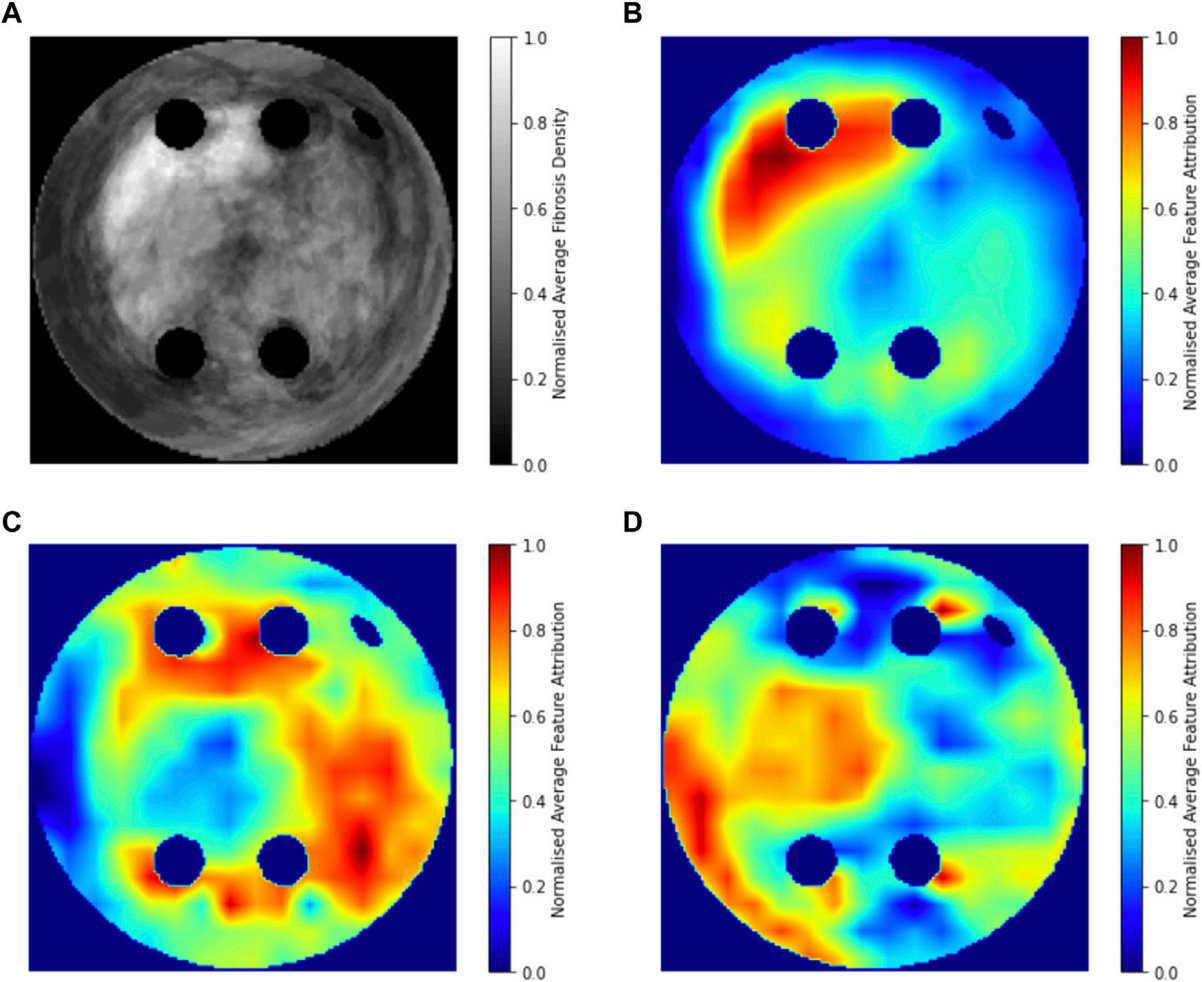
Averaged LGE MRI intensities and FA maps on the hold-out test set. (A). Averaged and normalised LGE MRI intensity in the LA tissue disks. (B). Averaged and normalised GradCAM FA map for the ROTOR ablation strategy. (C). Averaged and normalised GradCAM FA map for the FIBRO ablation strategy. (D). Averaged and normalised GradCAM FA map for the PVI ablation strategy.
